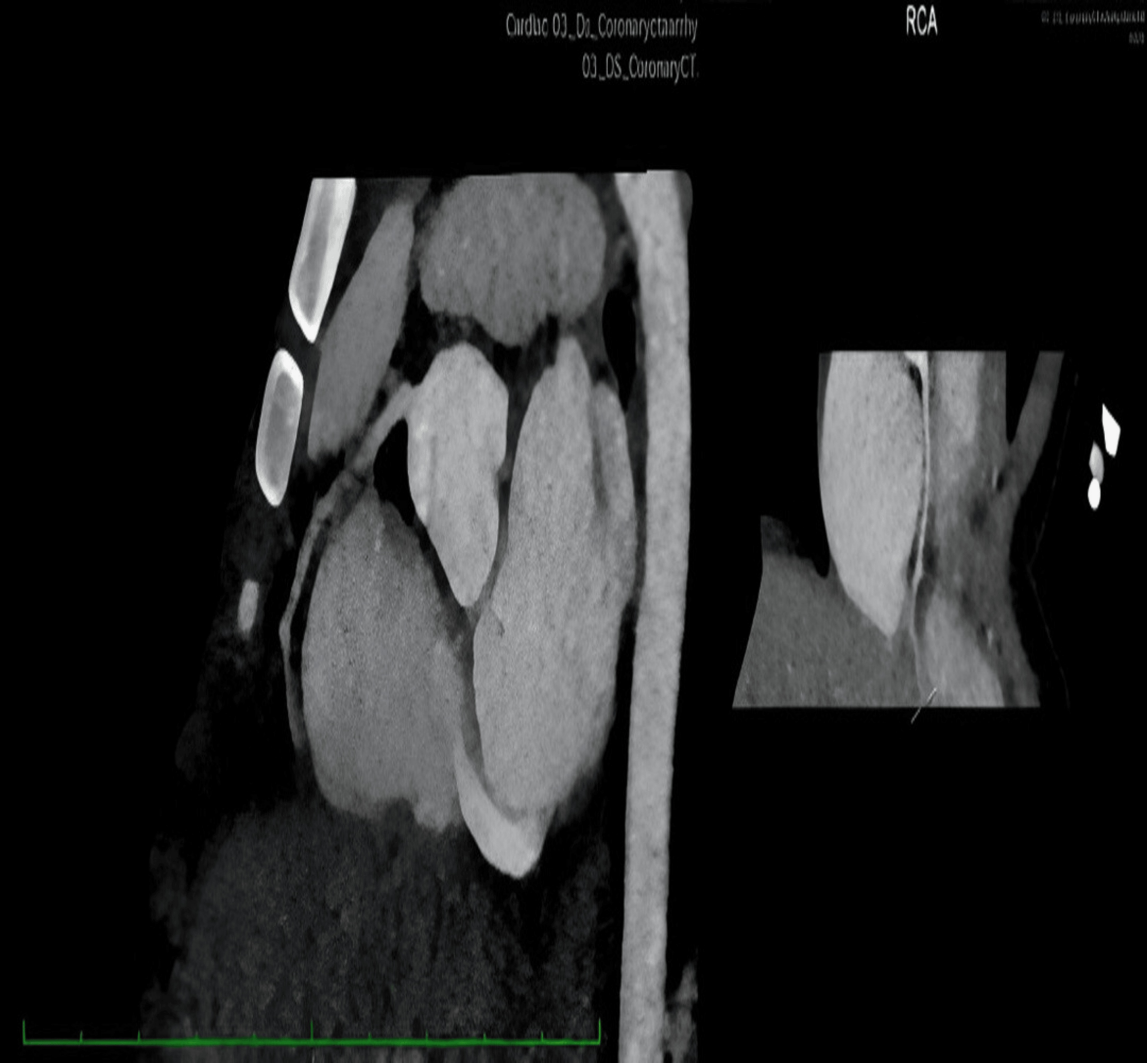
Computed tomography coronary angiography (CTCA) demonstrated regression of coronary arteries dilatation and resolved RCA aneurysm.
